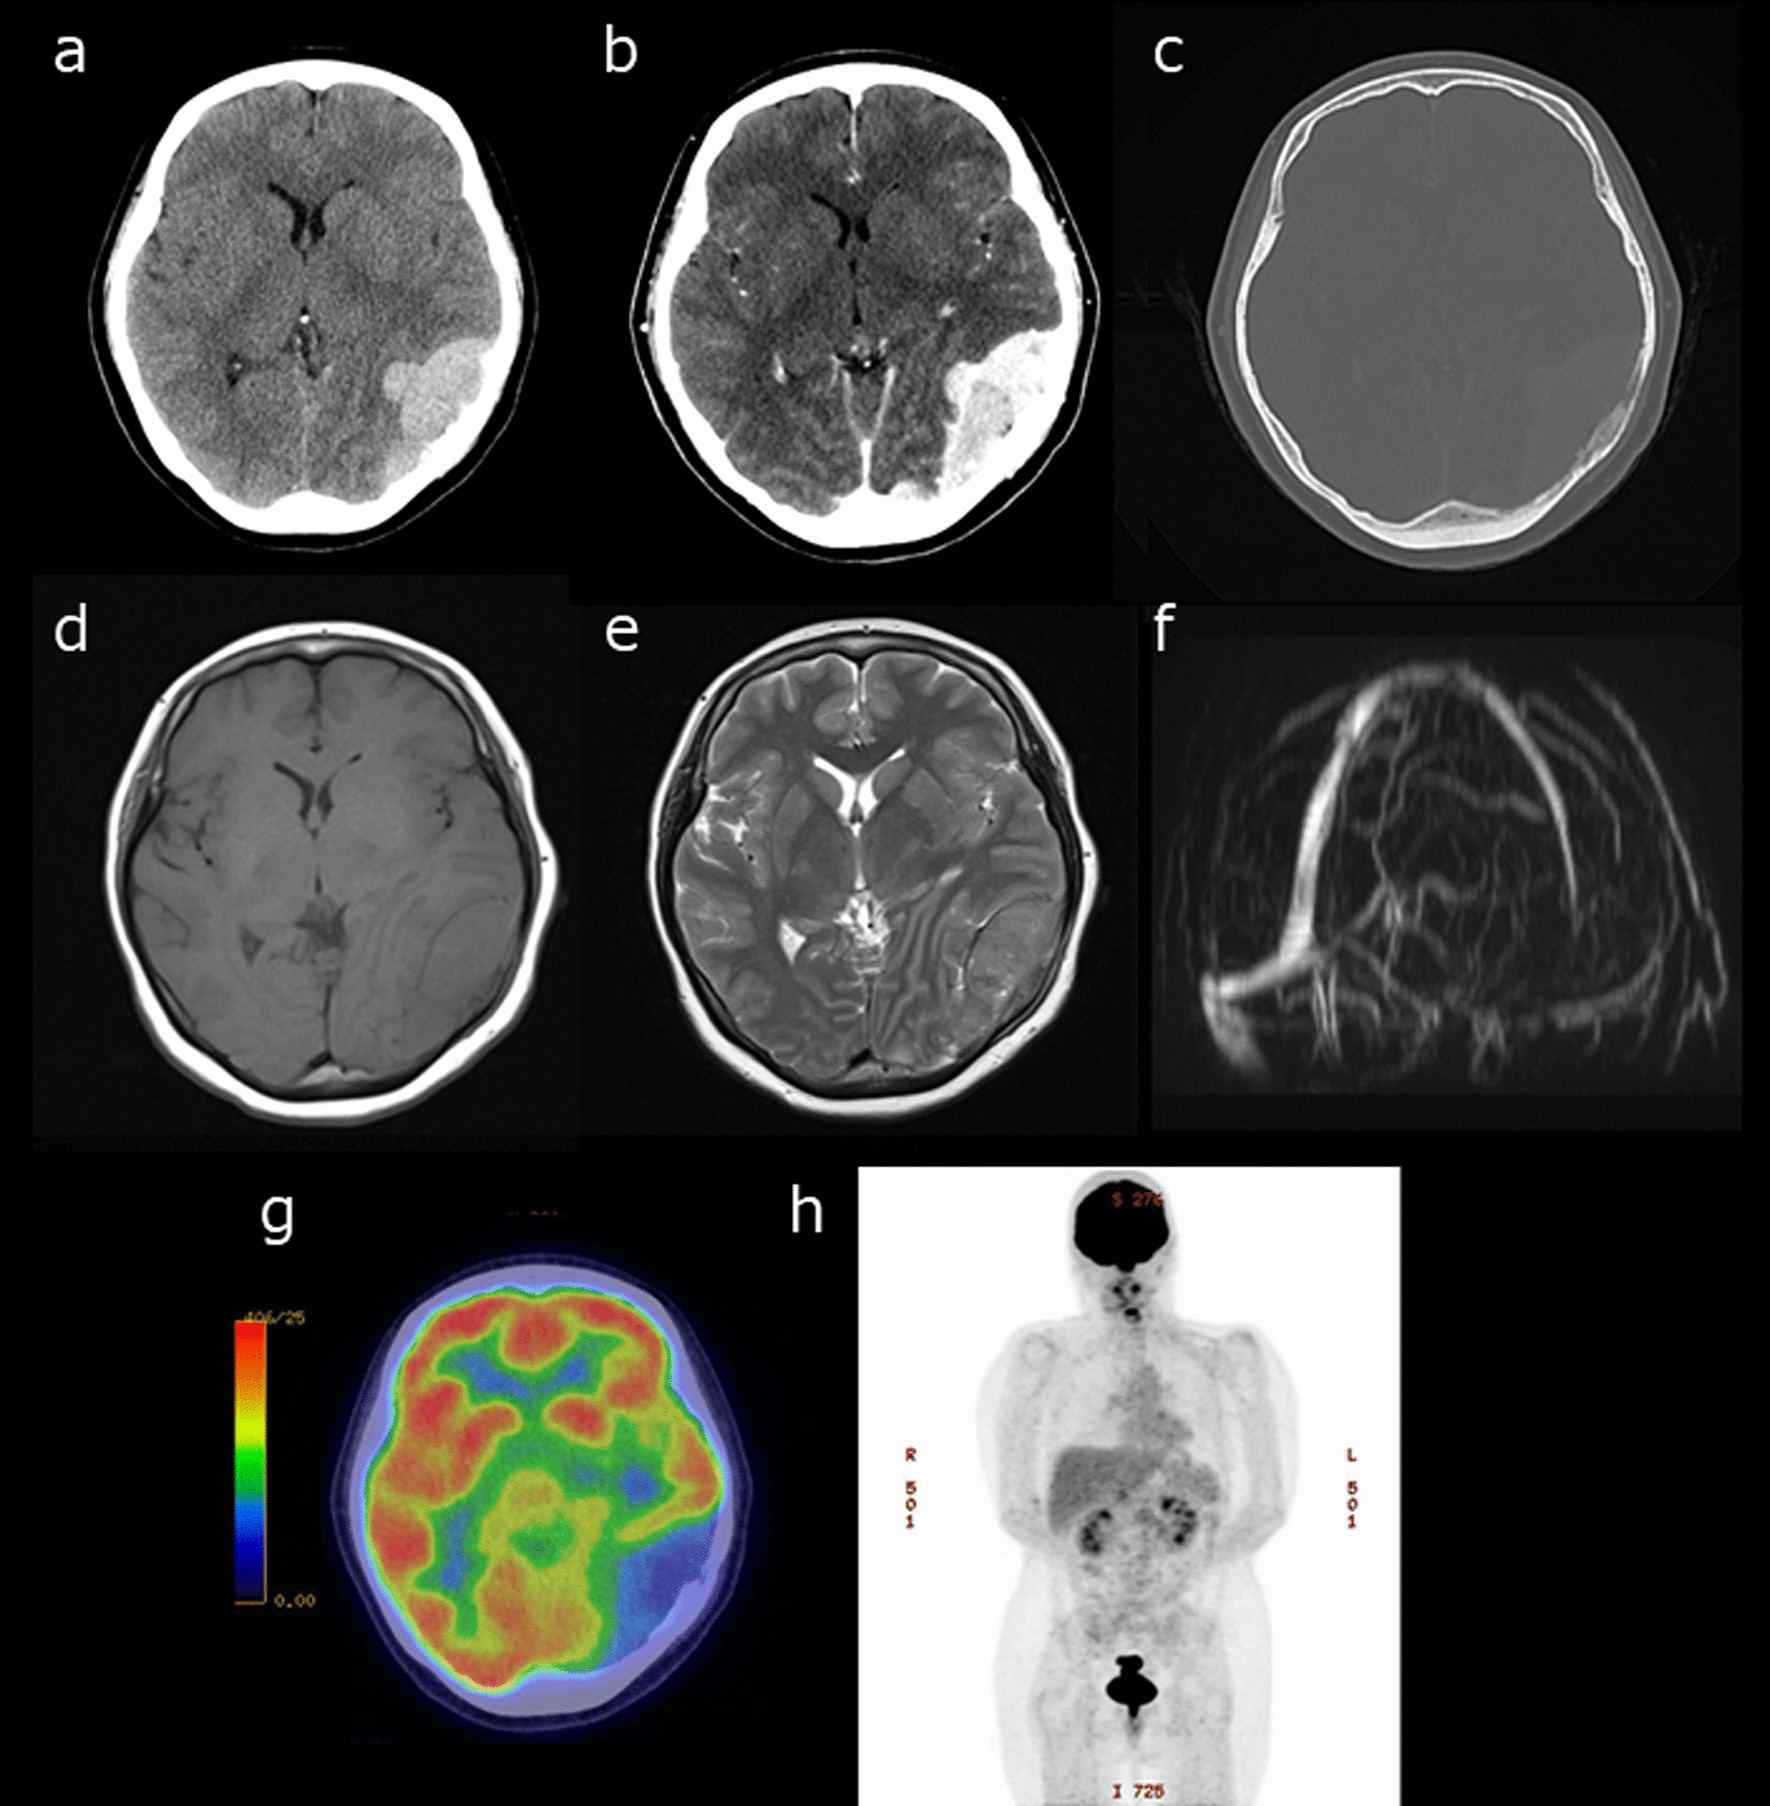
Precontrast head computed tomography (CT) shows a high-density lesion bordering the left occipital bone (a), and the contrast-enhanced head CT shows a contrast enhancement effect (b). Osteolytic changes are also observed in the left occipital bone (c). On the head magnetic resonance imaging (MRI), the lesion exhibits isodense signal intensity on T1-weighted imaging (d) and T2-weighted imaging (e). Magnetic resonance venography shows no signal in the left transverse sinus (f). ^18^F-fluorodeoxyglucose (^18^F-FDG) positron emission tomography-CT reveals low.^18^F-FDG uptake in the tumor (g) and no abnormally high uptake throughout the body (h)
